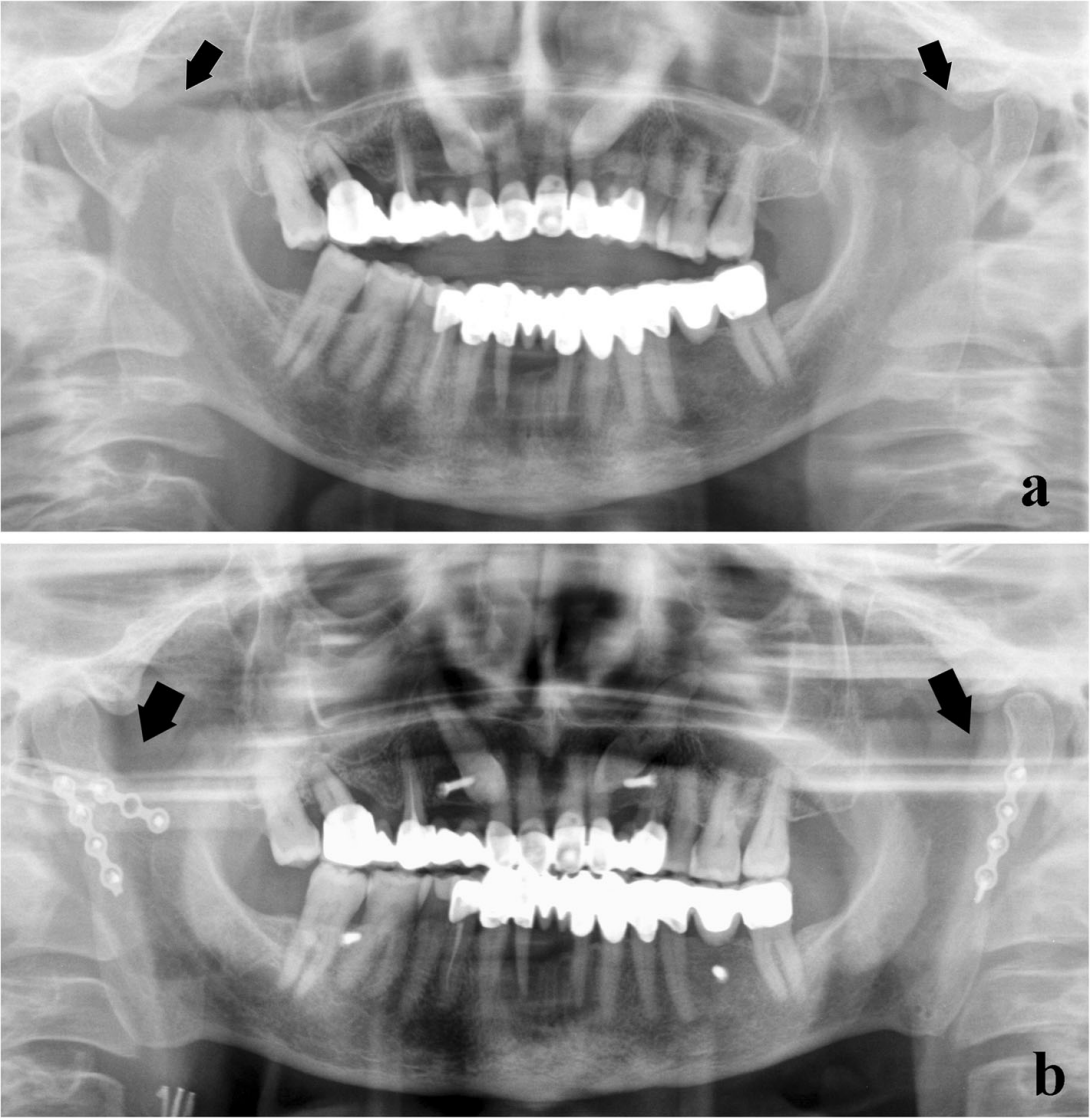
Bilateral condylar process fracture with extracapsular lateral dislocation. a Panoramic radiograph shows a fracture of both condylar necks (arrows), following a violent trauma on the chin symphysis. b The lateral displacement of the condylar heads was subjected to “open” reduction and rigid internal fixations with osteosynthesis plates (arrows)
